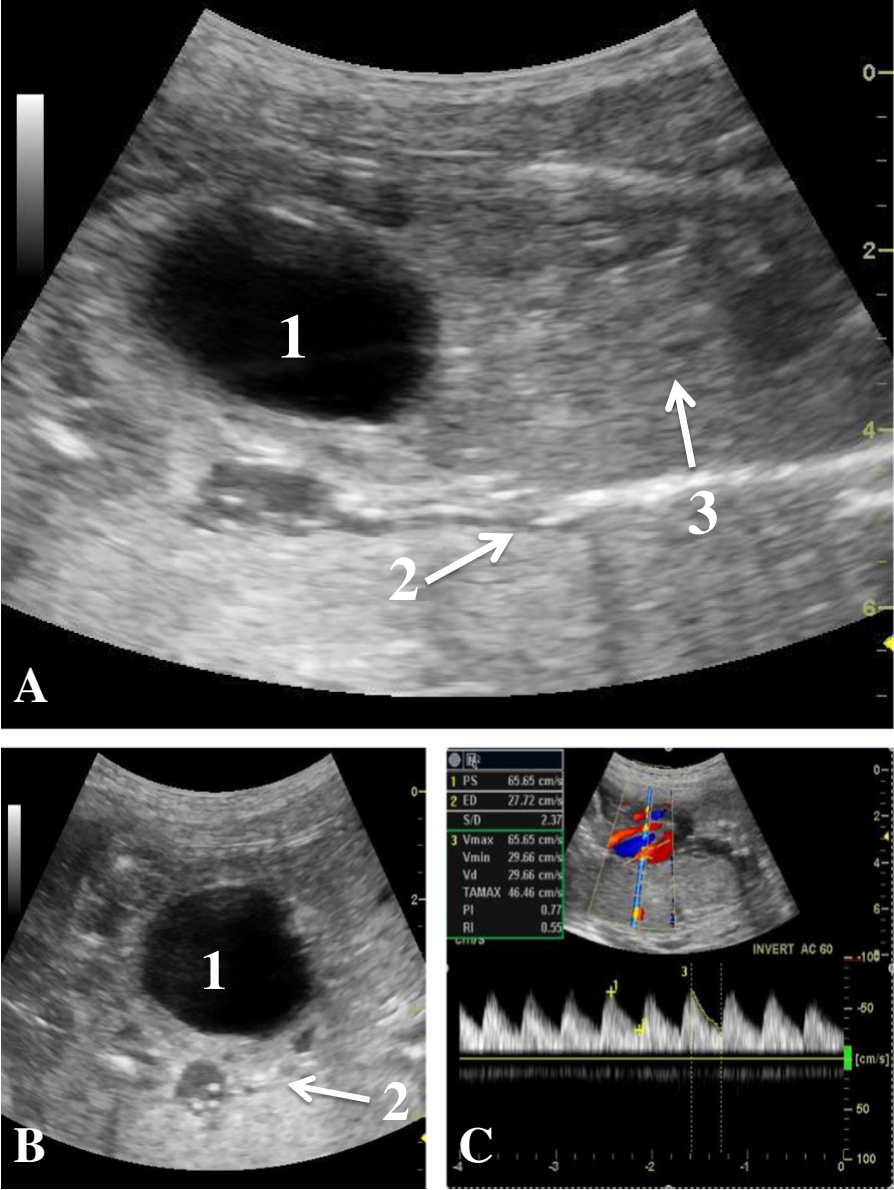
Prenatal ultrasound findings of a case of prune-belly syndrome in Papua New Guinea. A. Sagittal view – 1) megacystis, 2) anhydramnios, and 3) marked compression of abdominal and thoracic contents by the bladder. B. Coronal view - 1) megacystis and 2) anhydramnios. C. Umbilical artery Doppler at an estimated 22^+1^ weeks’ gestation.
